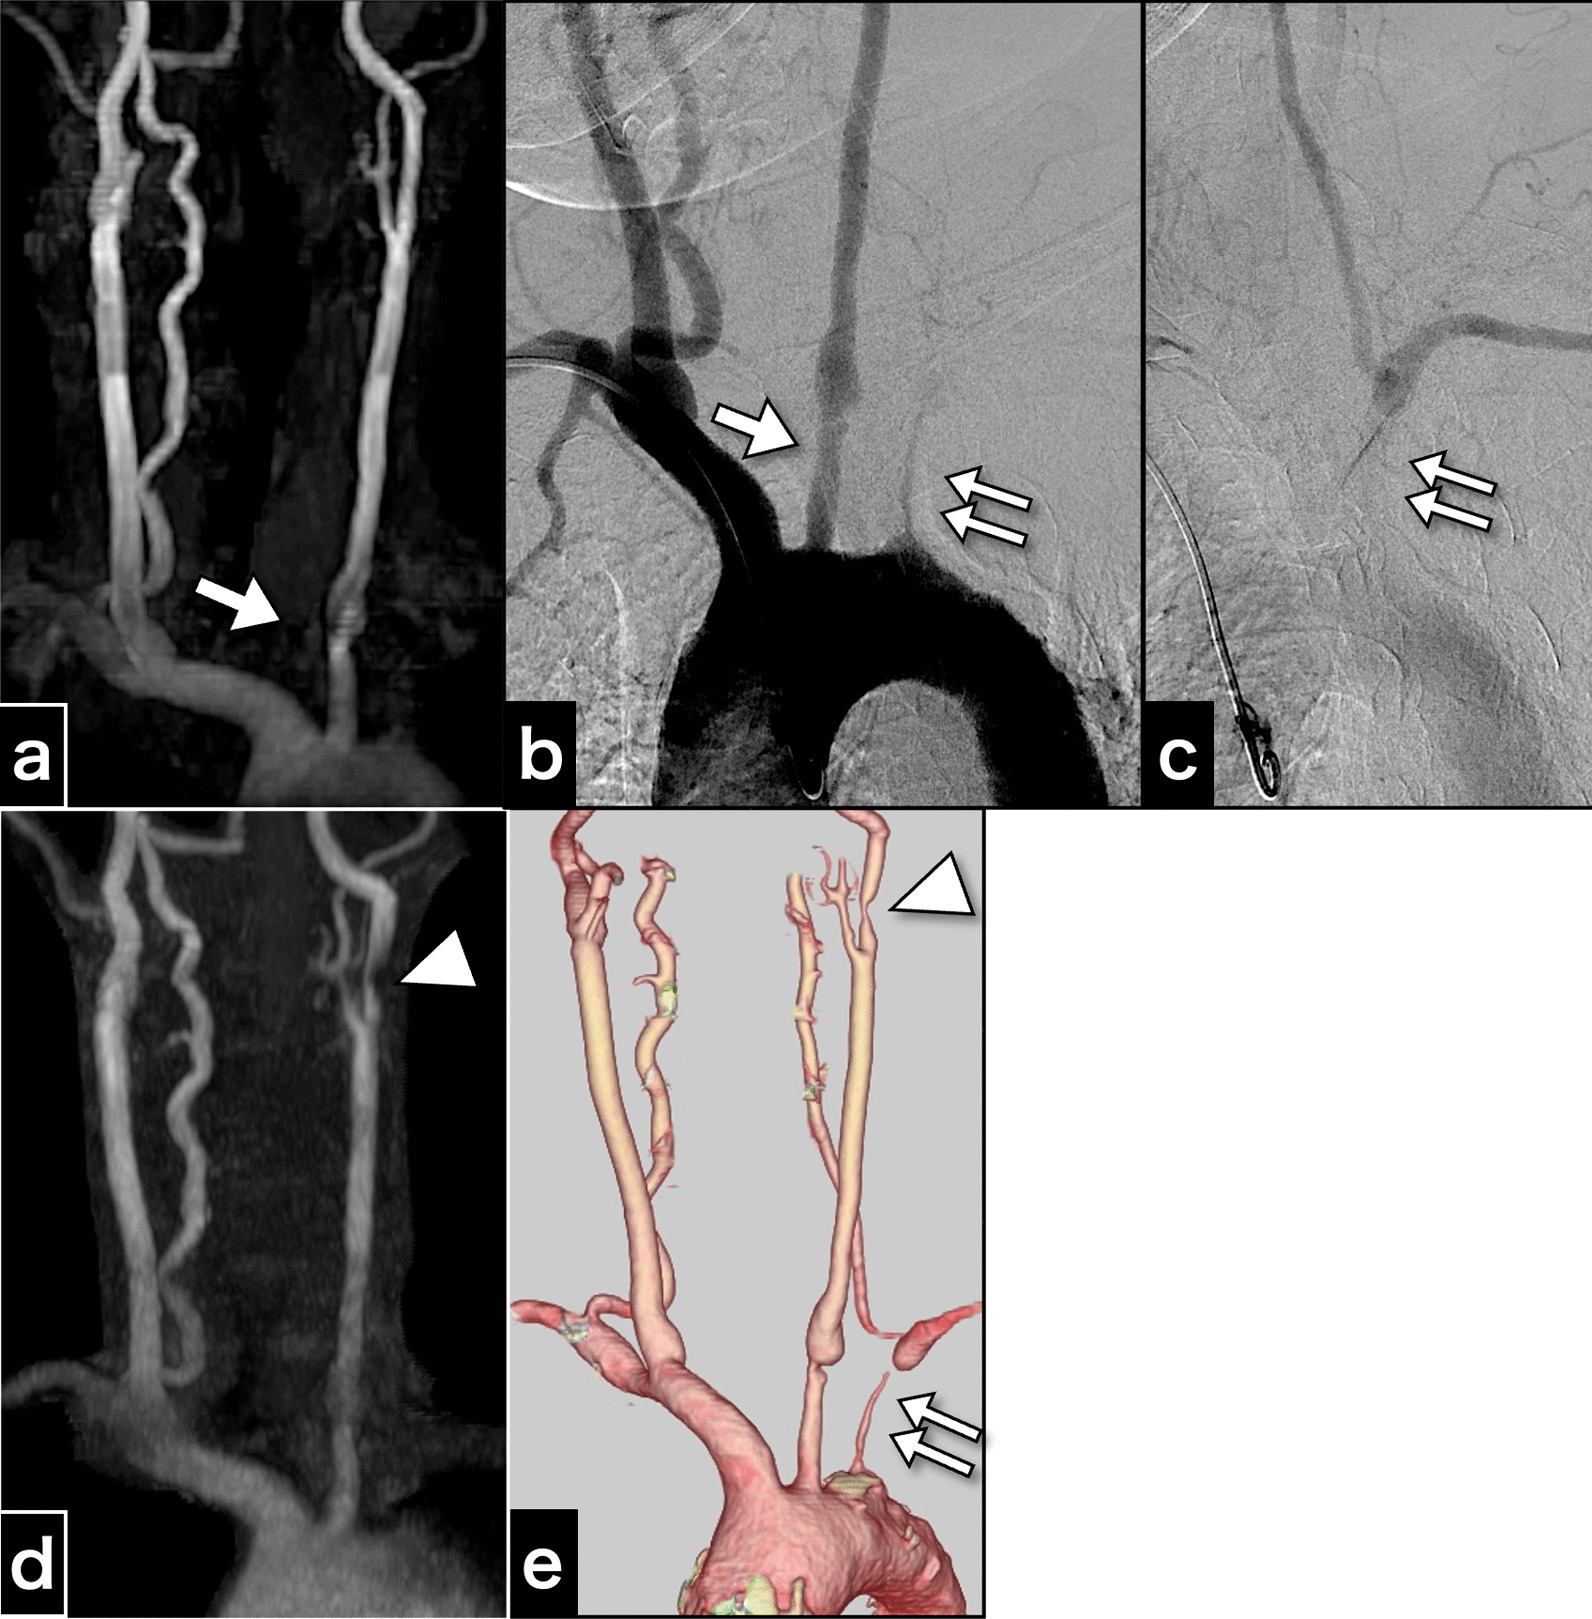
Cervical artery images obtained 3 years before carotid endarterectomy and just before carotid endarterectomy. a Magnetic resonance aortogram findings 3 years before carotid endarterectomy. b Aortogram findings 3 years before carotid endarterectomy. c The late-phase aortogram showing retrograde filling of the left vertebral artery and antegrade filling of the distal left subclavian artery. d Magnetic resonance aortogram findings just before carotid endarterectomy. e Computed tomography angiography findings just before carotid endarterectomy. The examinations performed 3 years before carotid endarterectomy a–c show an irregular origin of the left common carotid artery (arrow) and severe stenosis of the left subclavian artery (double arrow), and indications for the left subclavian steal phenomenon. The images just before carotid endarterectomy also depict progressive left internal carotid artery stenosis (arrowhead)
